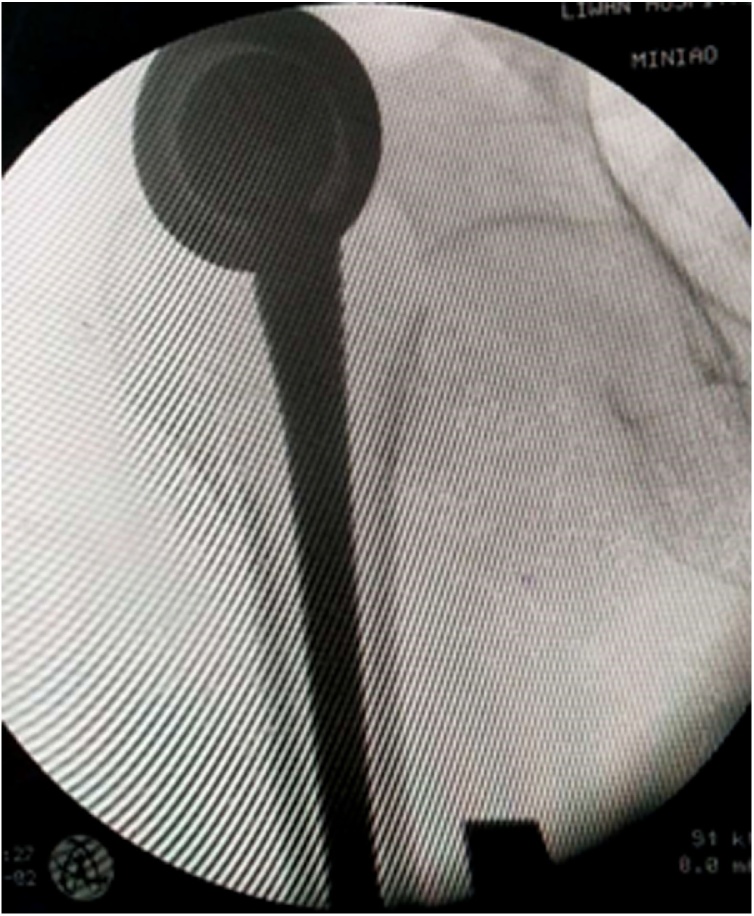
Intraoperative radiographs demonstrated dislocation still exists and preprosthesis fracture of femoral.
